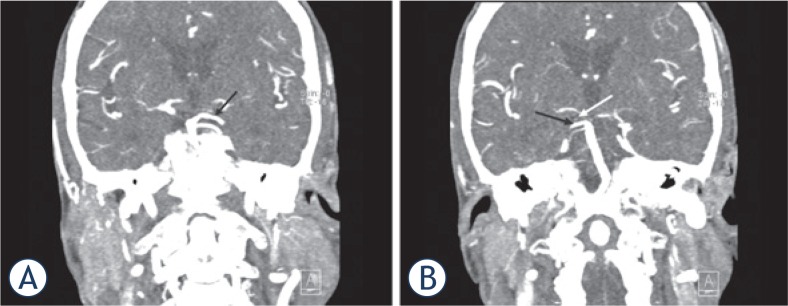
Computed tomography angiography (CTA) of the neck and intracranial arteries. The basilar artery and the left posterior cerebral artery (PCA) (black arrow) were both transient (A). At first glance, the right PCA appears to be fully opacified. Detailed examination of the CTA images revealed a filling defect of the P1 segment of the right PCA (white arrow) and another rare anatomic variant: duplication of the right superior cerebellar artery (black arrow), which could have been mistaken for a transient right PCA (B).
